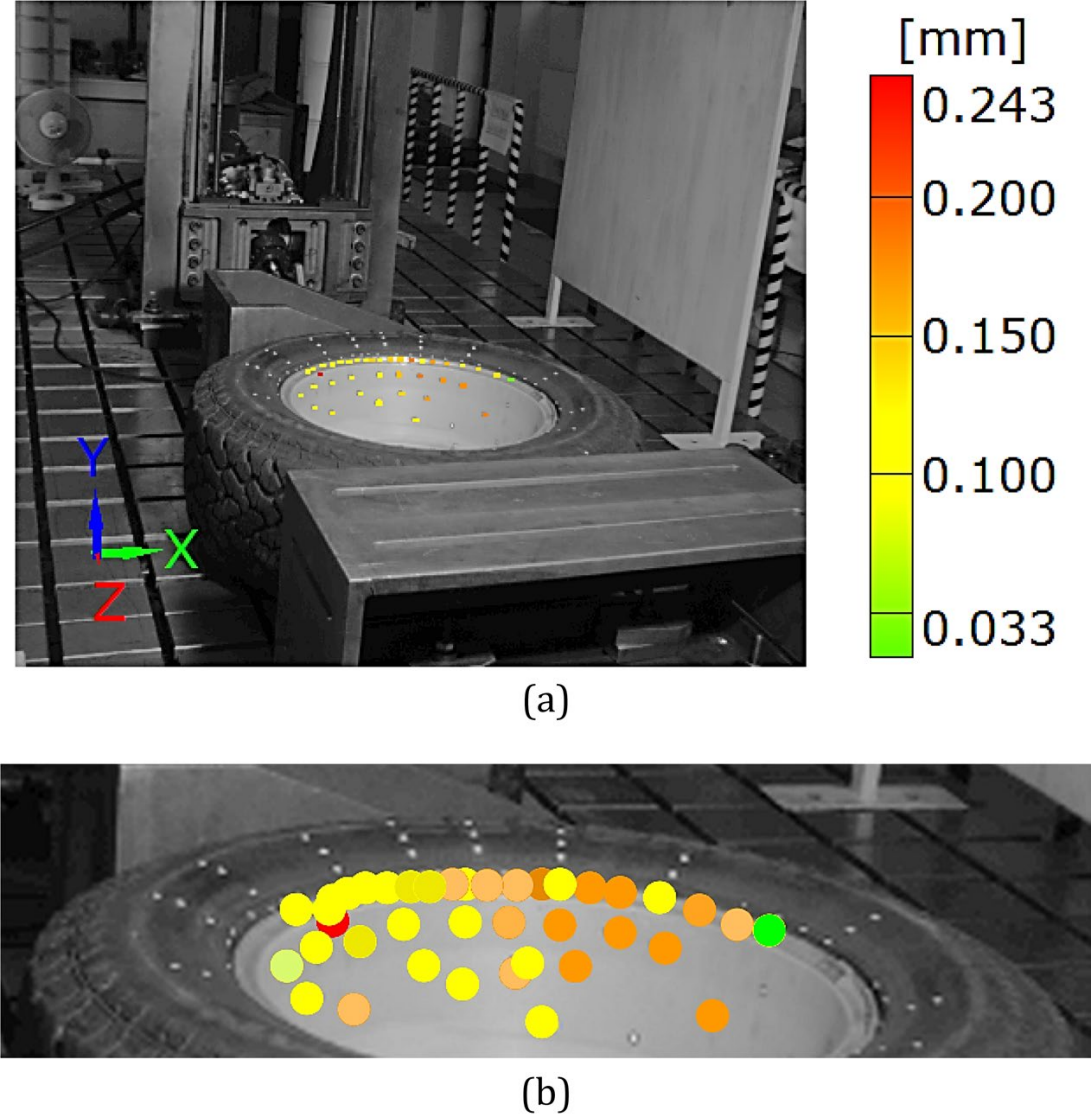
Distribution of the resultant vectors of deflection for the SUV rim after unloading: (a) general view, (b) magnified view of the tire.
